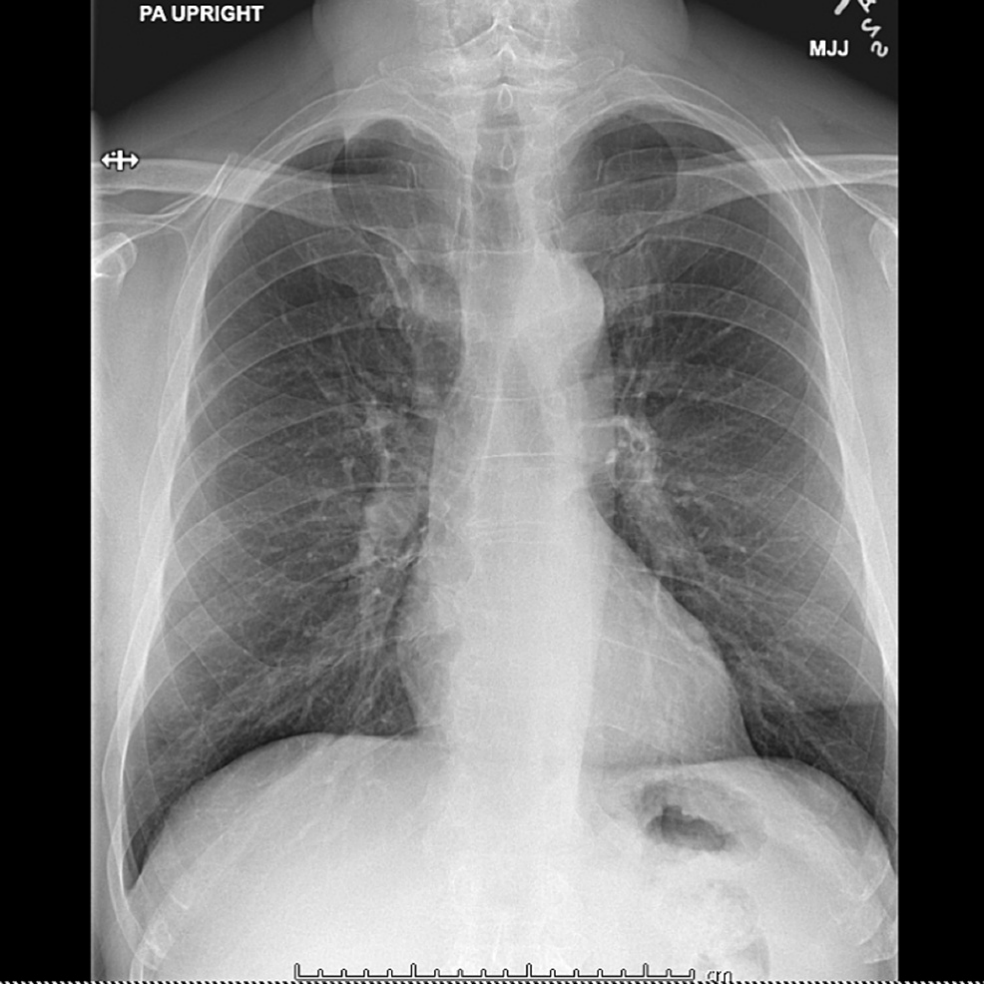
Chest x-ray of the patient shows hyperinflation without any other abnormalities
